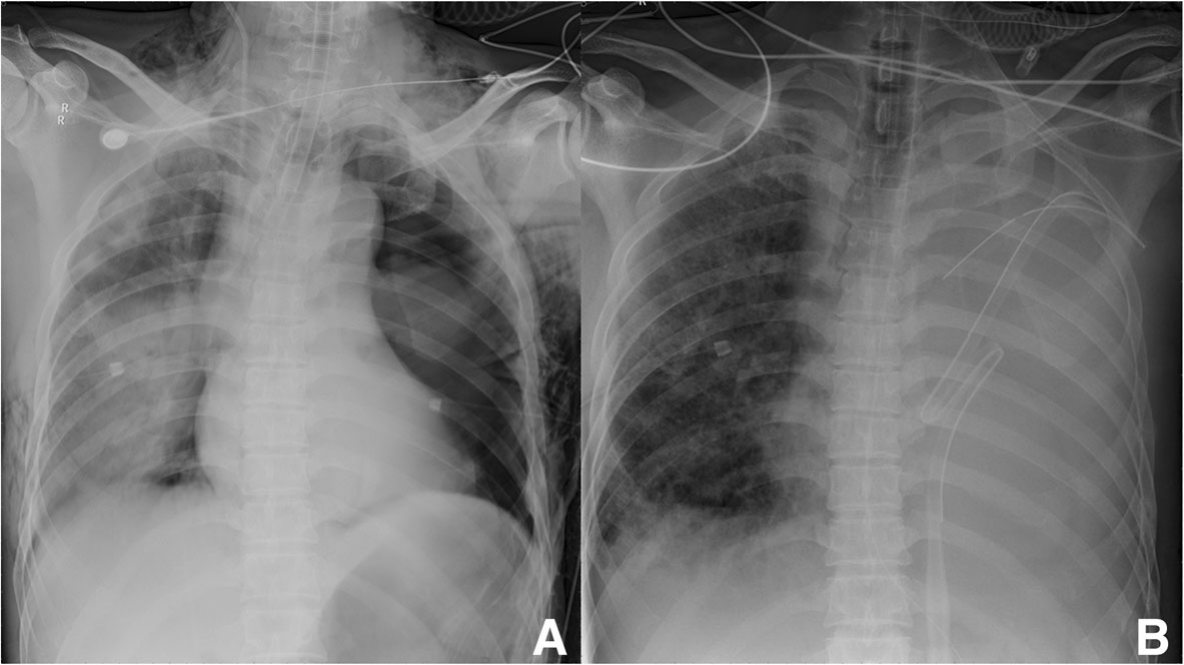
a was the x-ray taken on admission, there were bilateral pneumothorax, bilateral traumatic wet lung, and multiple rib fractures. b was the x-ray taken after ECMO was weaned, the condition of the right lung recovered significantly, edema and exudation alleviated; the left lung was removed
